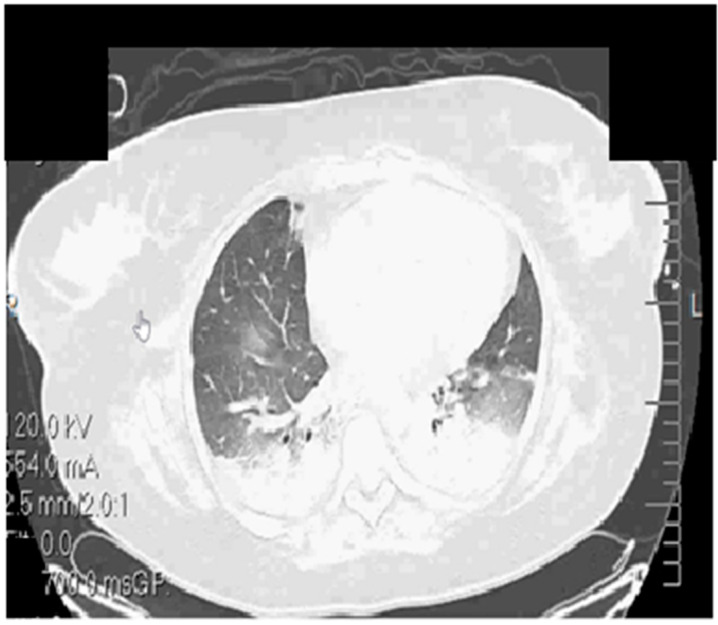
Native thorax CT at admission.
